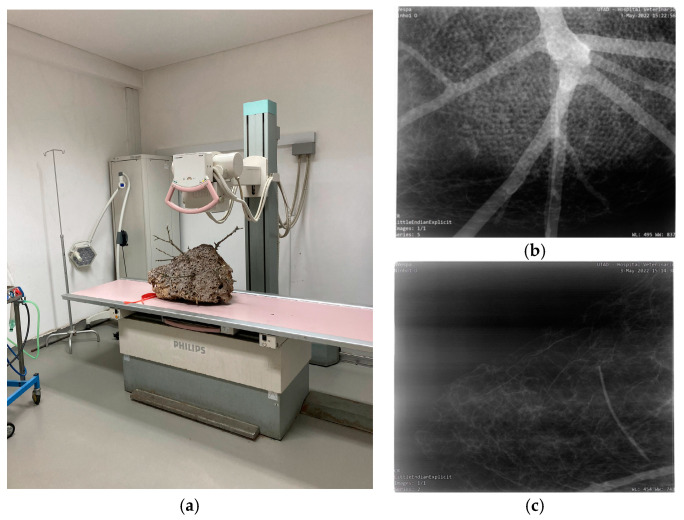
Radiographs of the AHN: (a) position of the nest during the X-ray scanning; (b) X-ray image of the tree branch and cells; (c) X-ray image of the outer envelope [47].
